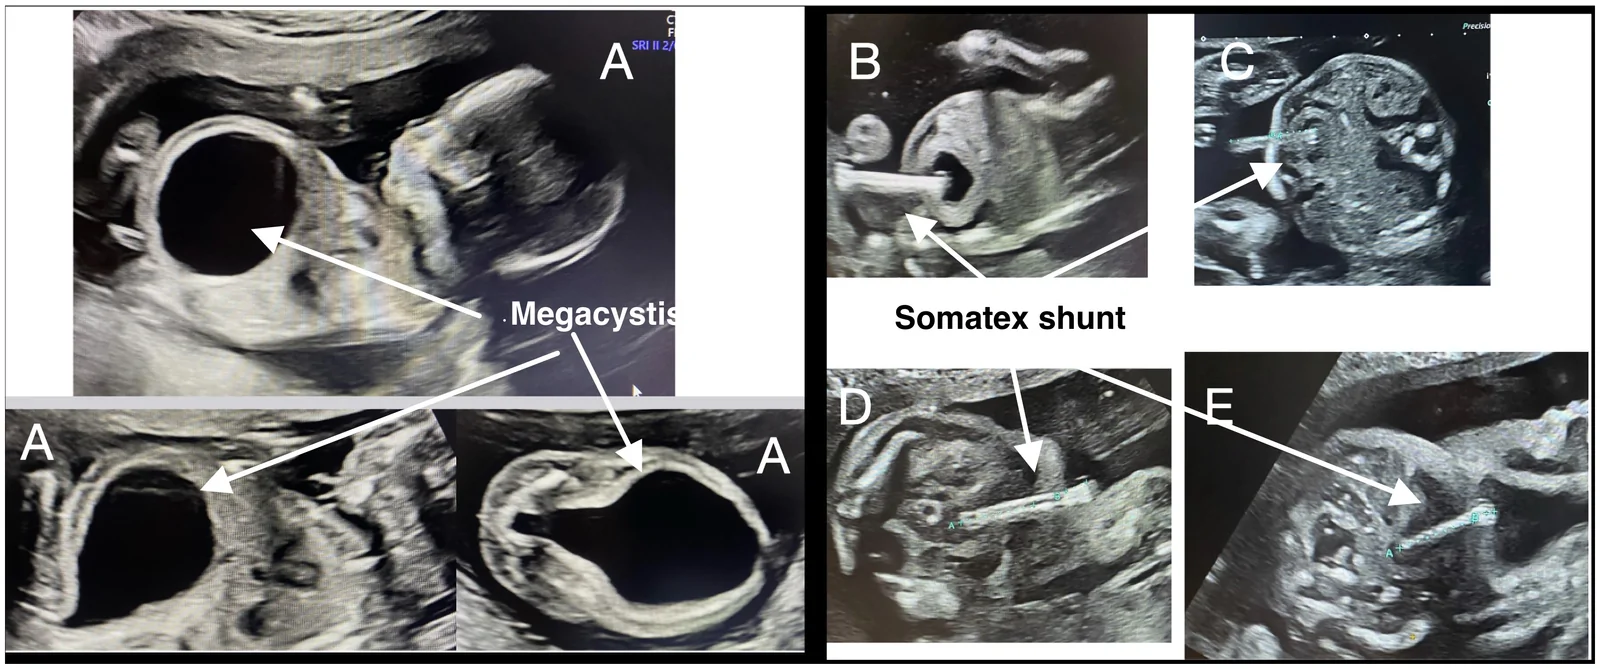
Fetal ultrasound. (A) Week 15 of gestation, megacystis (40 × 43 mm). (B) Week 16 with vesicoamniotic shunt. (C) Week 22. (D) Week 27. (E) Week 32.
